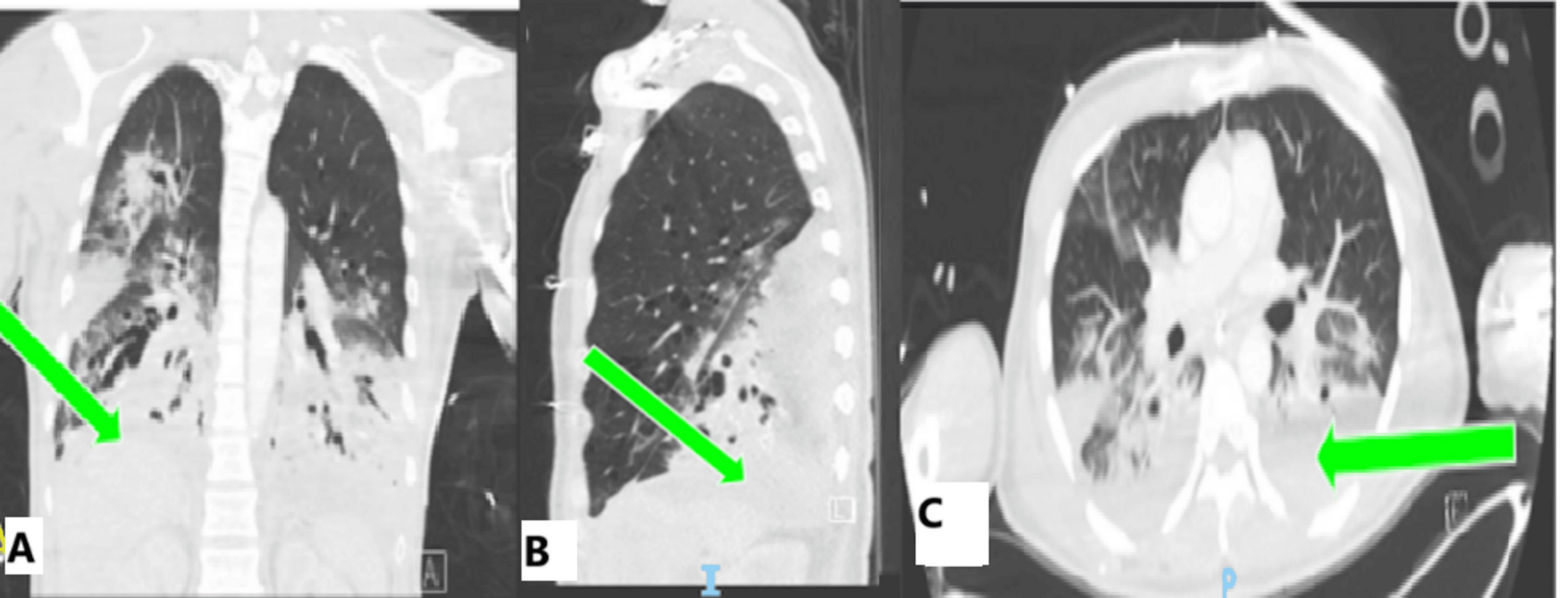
Computed tomography angiography of the chest and abdomen The computed tomography shows no signs of pulmonary embolism, small pleural effusions, no pneumothorax, and bilateral bronchiectasis. Also, there is extensive consolidation throughout all lobes, but the worst is in the lower lobes. Consolidation is shown in all planes: coronal (A), sagittal (B), and transverse (C)
